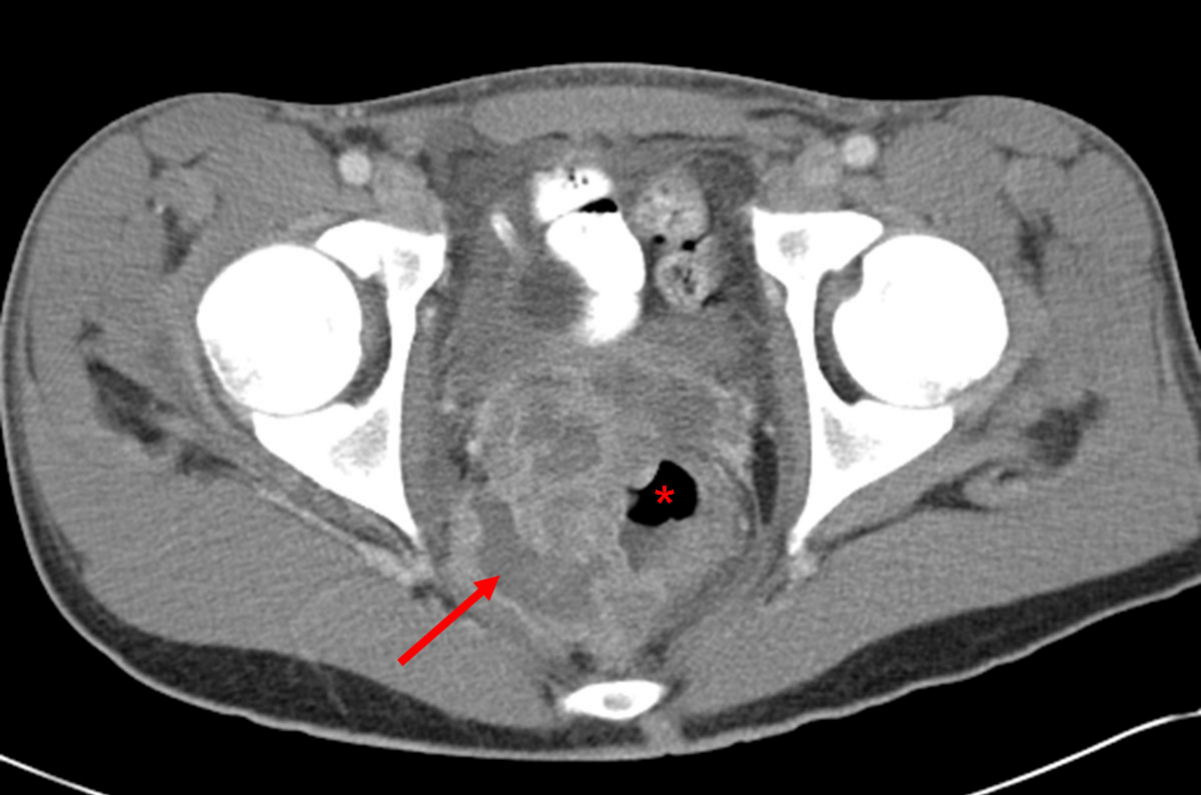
A computed tomography scan of the patient's abdomen and pelvis revealed an extensive, loculated fluid collection (arrow) encircling the distal rectum (*) with surrounding inflammation, consistent with a perirectal abscess. Additionally, a thickening of the wall of the terminal ileum with a fistulous tract from the distal terminal ileum to cecum was noted but is not shown in this figure.
